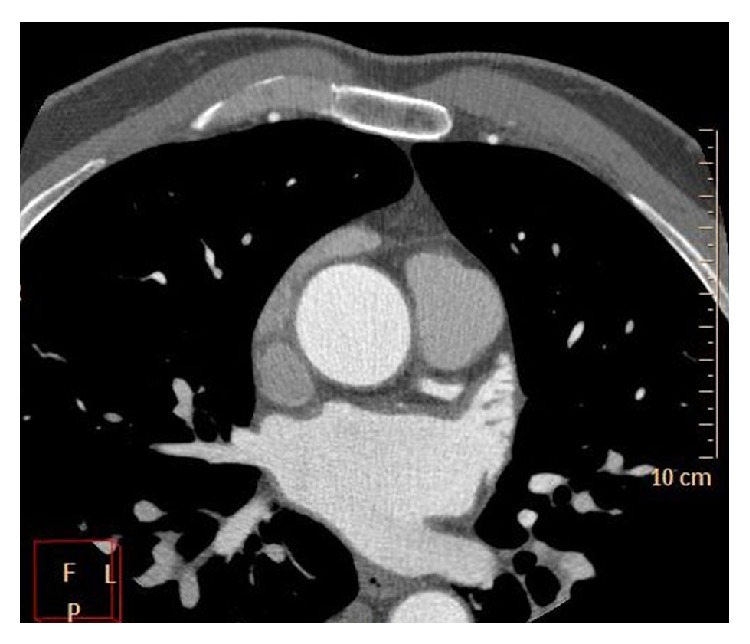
Contrast-enhanced CT image showing the most common subtype of left atrial appendage called chicken wing.
